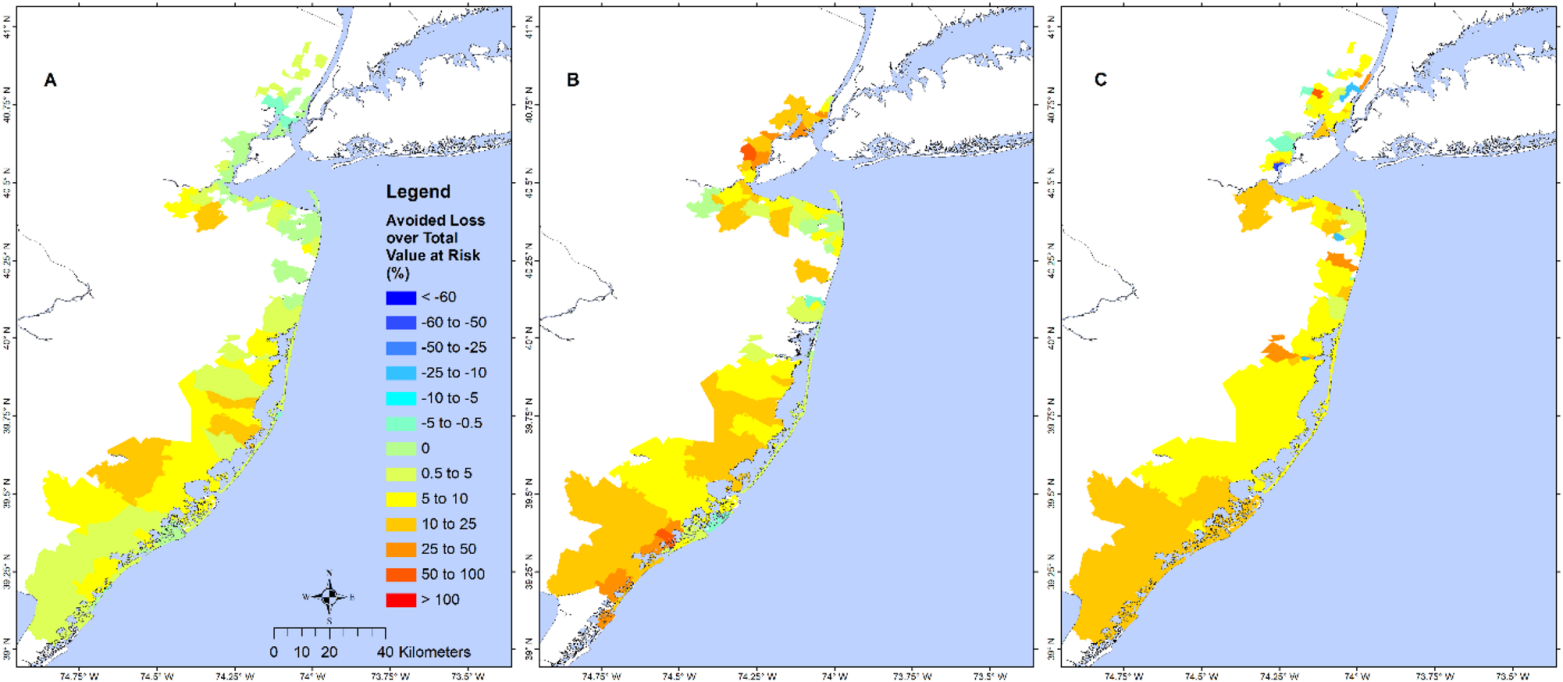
Wetland value at zip-code level. Map showing zip-code resolution avoided losses during (A) Sandy, (B) BS storm, and (C) 1% annual flood and wave, as a percentage of the total at-risk property value of the without wetlands scenario. Dark red values show zip-code with the highest wetland value while dark blue areas show the least wetland value. Negative values indicate that the presence of wetland would increase structural losses and positive values indicate that wetland would lower the structural losses. These maps show that wetland values for NJ are mostly between low (< 10%) and modest (10–25%). These maps, along with the wetland value regression model developed in this study, can be used by local planners to develop resiliency and restoration plans to increase the wetland coverage area and enhance the wetland value. Maps for NY and CT are unavailable due to unavailability of structural data there. The map is produced using ESRI ArcGIS Pro 2.7 (https://www.esri.com/en-us/arcgis/products/arcgis-pro/overview).
